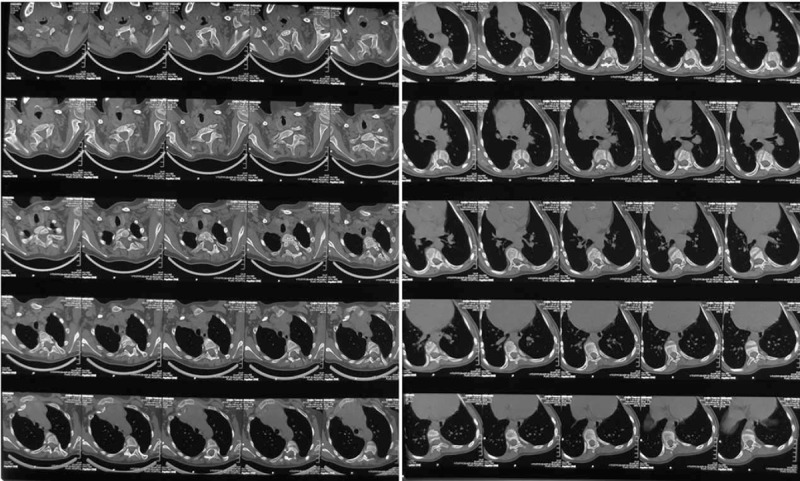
Computed tomography (CT) revealed a thoracic 5 and 6 vertebral body dysplasia.
